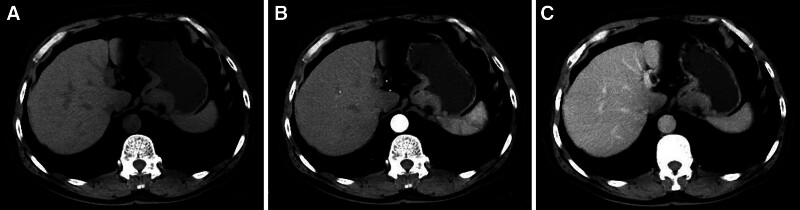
A typical case (male, 62 years old, microvascular/nerve invasion (-)) with local gastric wall thickening with abnormal enhancement on CT (T3 stage, N3 stage, adenocarcinoma, nonpoor differentiated, mixed type). (A) Plain CT image: local gastric wall thickening in the gastric cardia. (B and C) Enhanced CT image: the thickened gastric wall in the gastric cardia is significantly enhanced.
